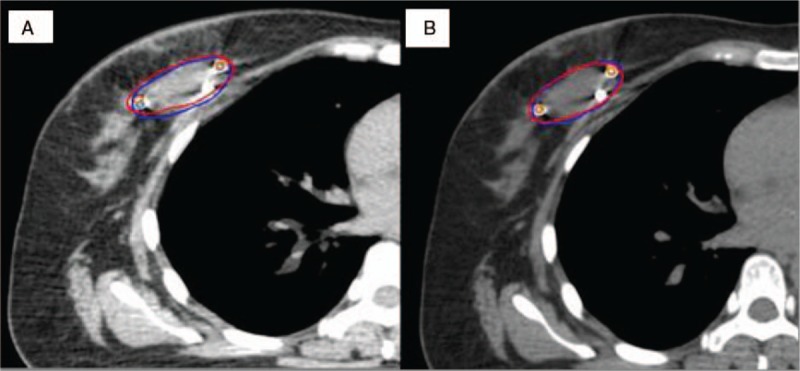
Original manual contour of the gross tumor volume at CT_50_ (blue) and the registered gross tumor volume at CT_0_ (red) using (A) rigid image registration and (B) deformable image registration. CT = computed tomography^^.
